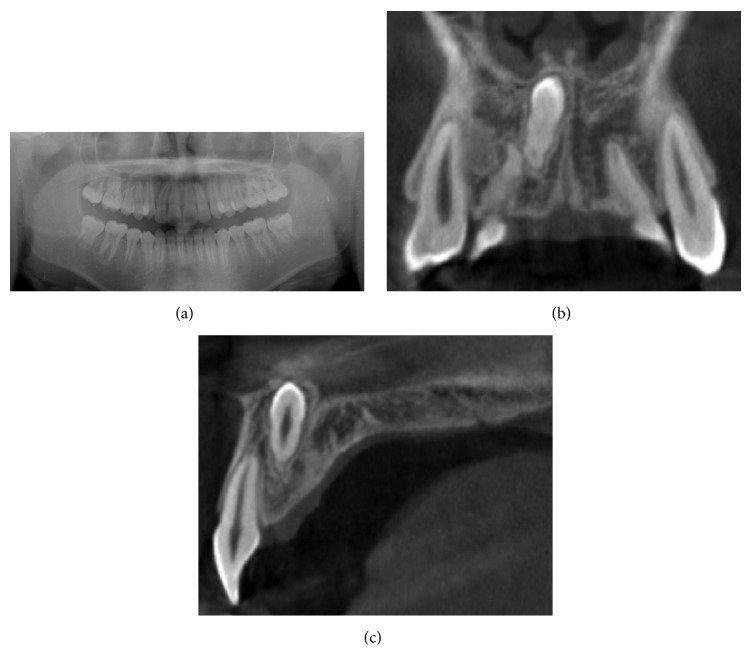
(a) Orthopantomograph X-ray and (b) coronal and (c) sagittal computed tomography images showing a deeply impacted supernumerary tooth in the anterior maxilla. The crown of the tooth was exposed on the nasal cavity side.
